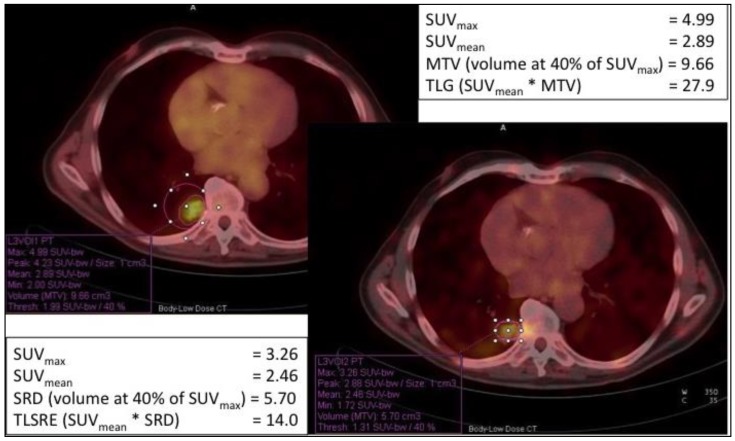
New evaluation prognostic parameters for NETs comparing [^18^F]-FDG PET/CT (left image) and [^68^Ga]-DOTA-NOC imaging (right image). The same lesion was analyzed by both [^18^F]-FDG and [^68^Ga]-DOTA-NOC, measuring SUV_max_, SUV_mean_ for both PET scans, MTV and TLG for [^18^F]-FDG, SRD and TLSRE for [^68^Ga]-DOTA-NOC. Considering the same volume of lesion, TLG and TLSRE values reported differential expression of SSTR and glucose transporters (GLUT) in the lesion. SUV = Standard Uptake Value; MTV = Metabolic Tumor Volume; TLG = Total Lesion Glycolysis; SRD = Somatostatin Receptor Density; TLSRE = Total Lesion Somatostatin Receptor Expression.
